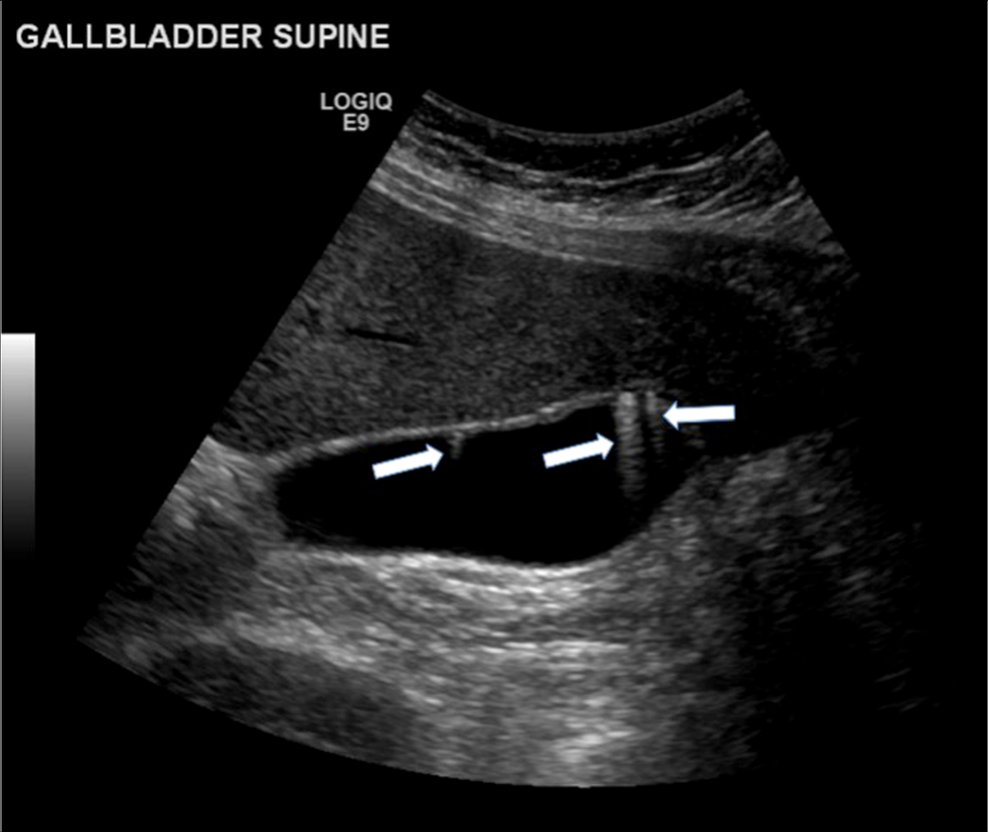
Pseudopolyps. This selected image from a transabdominal ultrasound scan demonstrates three separate pseudopolyps. Note the reverberation or “comet-tail” artefact posterior to the lesions (*arrows*)
